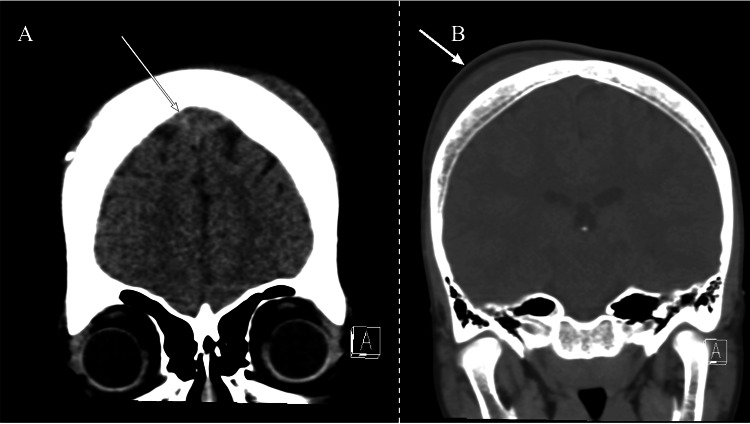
Coronal CT of epidural and subgaleal hematomas. Epidural (A) and subgaleal (B) hematomas are indicated by arrows.
